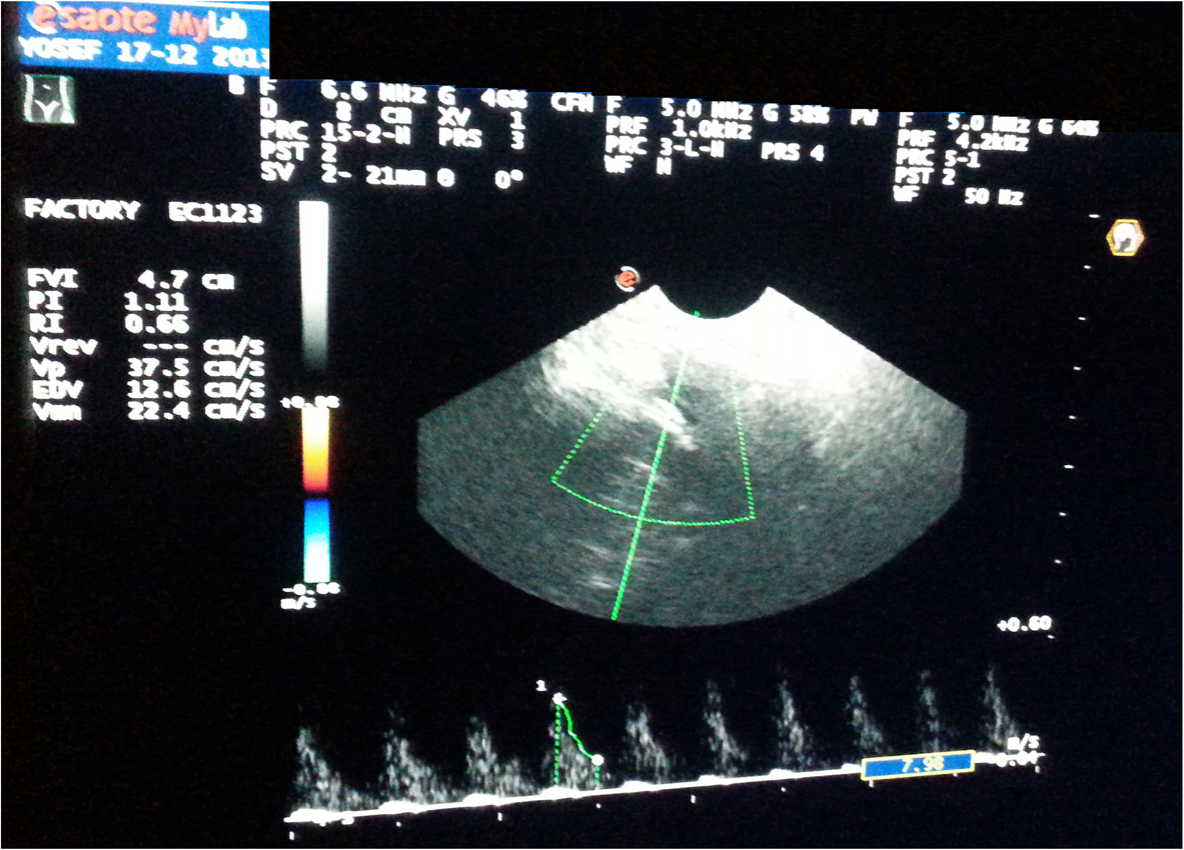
Colored Doppler Ultrasonography after KMC.
